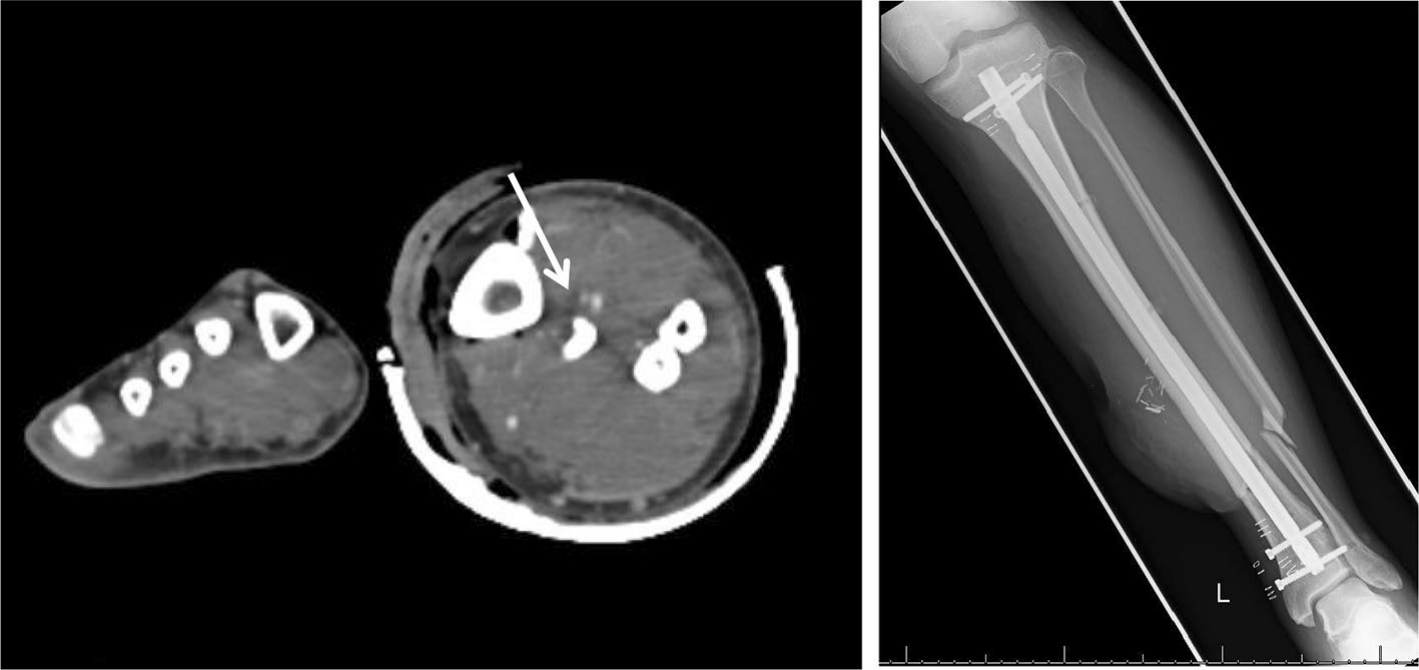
Radiograph of an open tibial fracture and CTA showing associated vascular injury to anterior tibial artery
